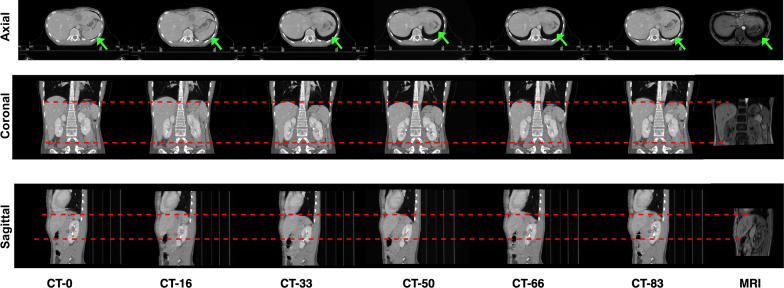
Coronal, axial and sagittal views of a patient’s 4D-CT volumes over the respiratory cycle (6 phases) and companion auxiliary breath-held MRI on expiration (50%). Notice the large respiratory motion across CT phases, as well as contrast differences and field-of-view discordance between CT and MRI.
